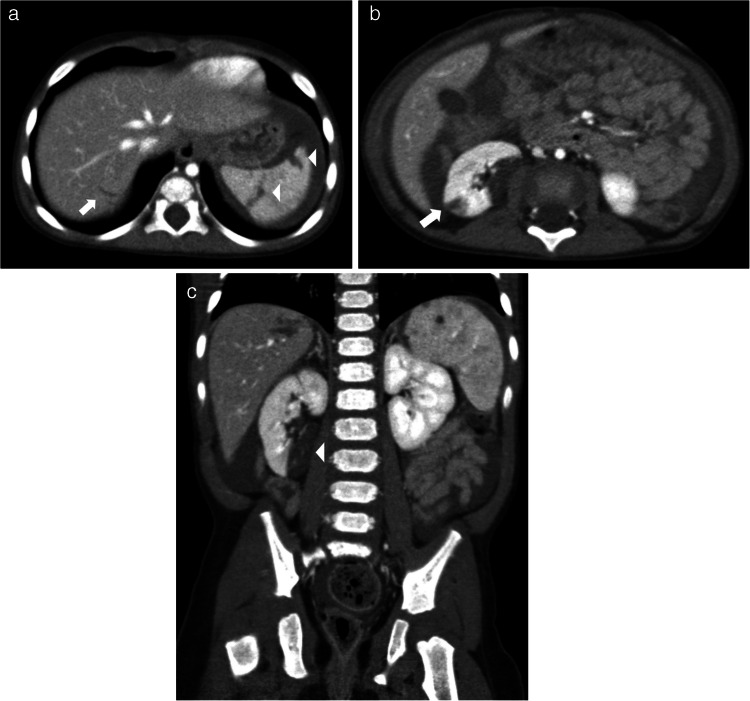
A 17-month-old male presented with lethargy, vomiting, extensive facial and neck bruising, and subconjunctival hemorrhages, concerning for physical abuse. a CT abdomen pelvis in portal venous phase shows grade II splenic (*white arrowheads*) and liver (*white arrow*) lacerations. b Axial image demonstrates a right renal laceration (*white arrow*). c Coronal image of the same study shows segmental devascularization injury of the renal lower pole (*white arrowhead*). Ascites as well as previously noted hepatic and splenic lacerations are also visible in the image (*unmarked*)
